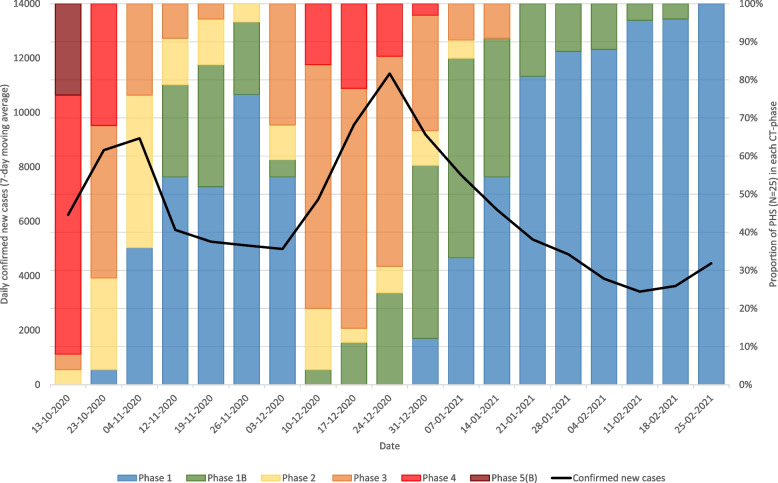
Daily confirmed new COVID-19 cases and proportion of Dutch public health services in each CT-phase. Based on contextual data regarding the weekly CT-phases of Dutch PHS that were collected and provided by each respective PHS and were shared with the Dutch National Institute for Public Health and the Environment (RIVM) by the umbrella organization of PHS in the Netherlands (GGD GHOR NL) and publicly available data regarding the daily number of confirmed COVID-19 cases in the Netherlands [10]
